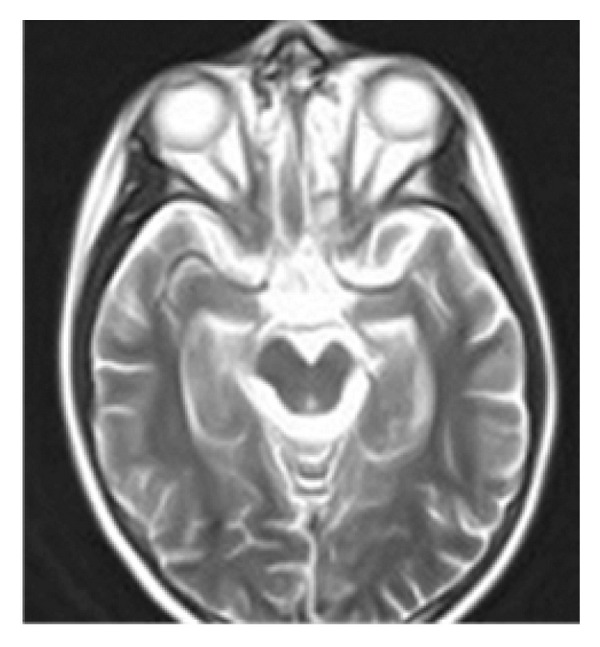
T2-weighted images reveal an atrophic left midbrain peduncle.
